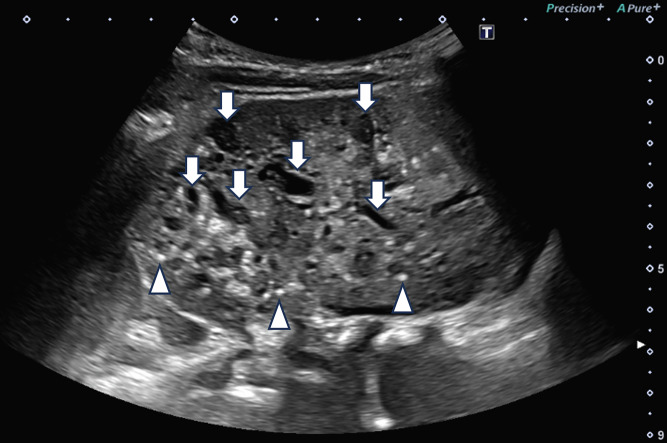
Ultrasound image of left kidney at 1 year of age shows renal enlargement (maximum diameter 96 mm), multiple cysts (with larger ones indicated by arrows) and numerous hyperechoic foci (some marked with arrowheads).
